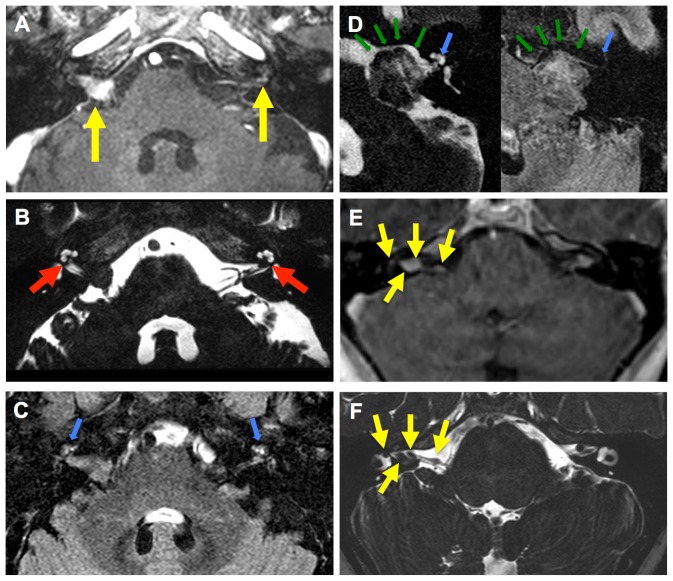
Magnetic resonance imaging in patients with neurofibromatosis type 2. Magnetic resonance (MR) imaging performed in a 47-year old patient with neurofibromatosis type 2 (NF2): (A) Axial, contrast enhanced, T1-weighted, magnetic resonance imaging demonstrates bilateral cochleovestibular schwannomas (CVS) (yellow arrows). (B) T2-VISTA sequence identifies the presence of a cerebrospinal fluid obstruction between the posterior fossa and the cochlear aperture (red arrows) bilaterally, independent of tumor size. (C) Pre-contrast fluid attenuated inversion recovery (FLAIR) sequence demonstrates the presence of elevated protein within the labyrinth (blue arrows). MR-imaging performed in a 48-year old patient with NF2: (D) Axial T2-VISTA and pre-contrast FLAIR sequences demonstrate the presence of normal labyrinthine protein (blue arrow) in the setting of a large CVS with an open CSF pathway (green arrows) between the posterior fossa and the cochlear aperture. MR-imaging performed in a 8-year old patient with NF2. (E) Axial, contrast enhanced, T1-weighted, magnetic resonance imaging demonstrates bilateral cochleovestibular schwannomas (CVS) with multiple tumors present on the right (yellow arrows). (F) T2-VISTA sequence identifies 4 discrete tumors, including a discrete intralabyrinthine tumor (yellow arrows).
